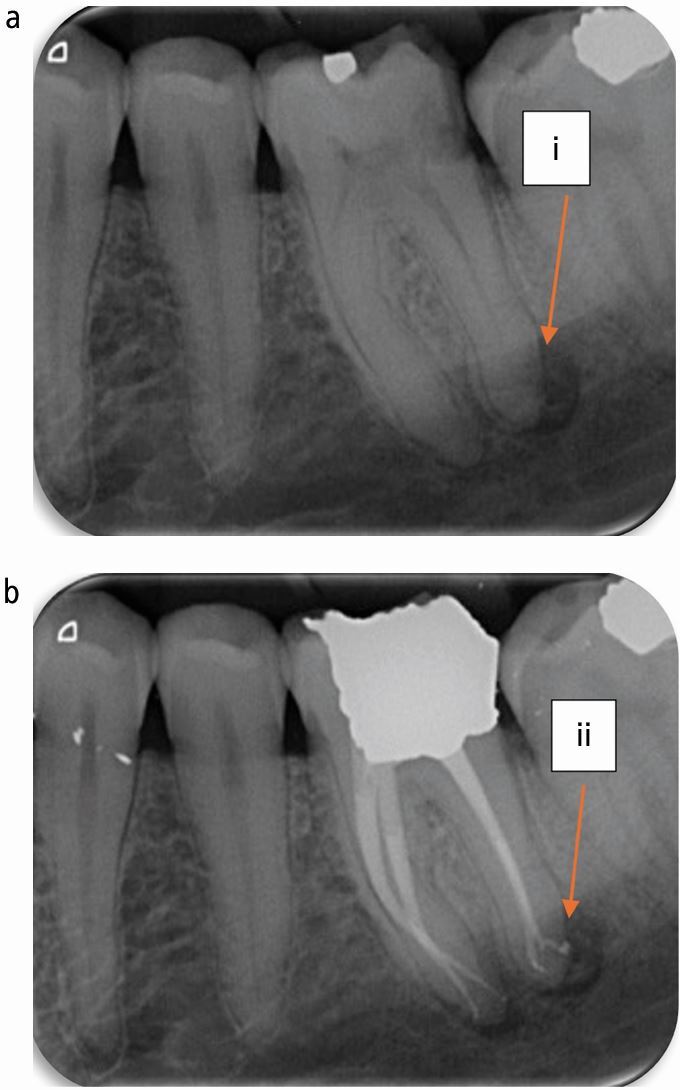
Position of the periapical lesion indicating the position of the portal of exit of the canal. a) Periapical radiolucency lateral to the radiographic apex of the root (i). b) Portal of exit of canal lateral to the radiographic apex and central relative to the periapical radiolucency (ii)
